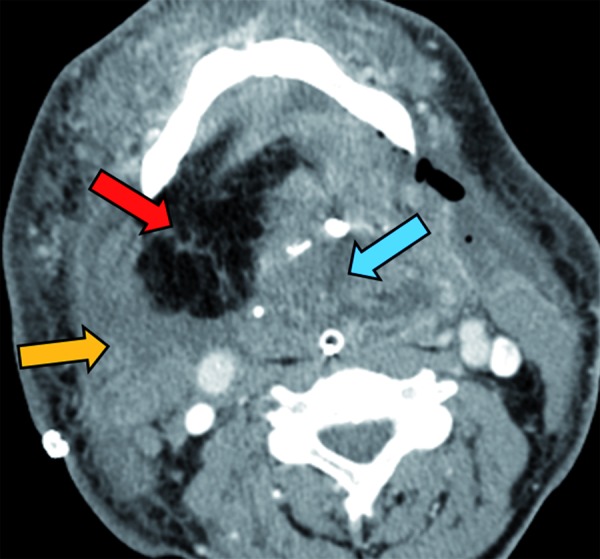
Post-operative CT-scan. Reconstruction of the resected floor of the mouth and bilateral cervical radical dissection with a musculocutaneous pedicled pectoralis major flap. Note the muscular component of the flap (orange arrow) and the fat tissue component (red arrow) covering the entire floor of the mouth. Remnant 1/3 posterior tongue is signaled with a blue arrow.
